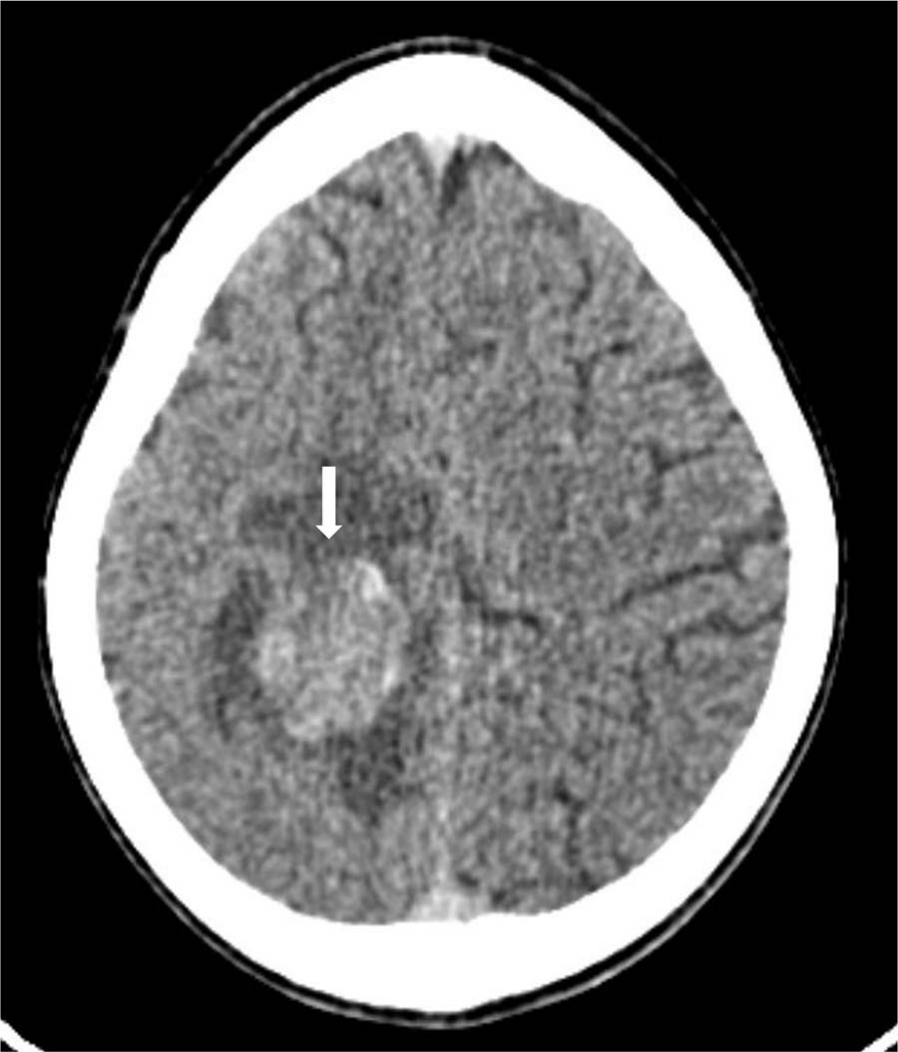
Axial brain CT scan showing an ovoid, well-defined right parietal lesion (arrow) with heterogeneous hyperdensity, surrounded by perilesional edema.
